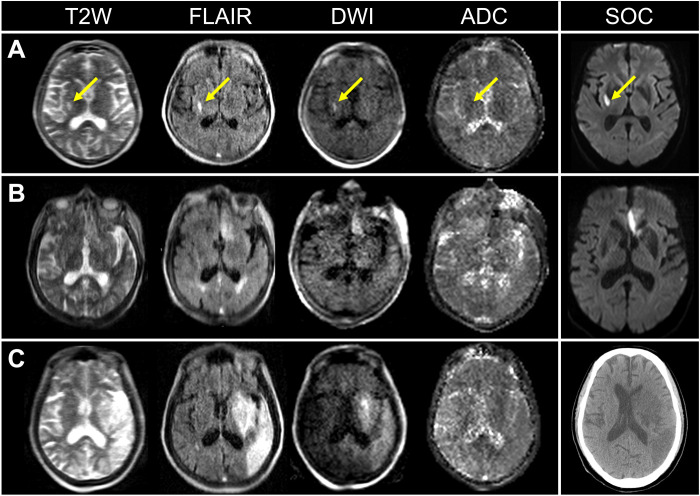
Ischemic infarcts of various lesion sizes shown on pMRI. (A) A 75-year-old female with a right middle cerebral artery infarct (13 mm in diameter) (yellow arrows) imaged by pMRI and SOC MRI (3 T; FLAIR) (30 and 48 hours since last known normal, respectively). (B) A 91-year-old female who received mechanical thrombectomy for the removal of a left anterior cerebral artery thrombus at 3 hours since last known normal. Subsequent pMRI and SOC MRI exams 13 and 19 hours after the neurointervention, respectively, detected a left anterior cerebral artery infarct (32 mm in diameter). (C) A 69-year-old male with a left middle cerebral artery infarct (114 mm in diameter) imaged by pMRI and SOC computed tomography (114 and 104 hours since last known normal, respectively). SOC exams included MRI (DWI sequence) and computed tomography.
